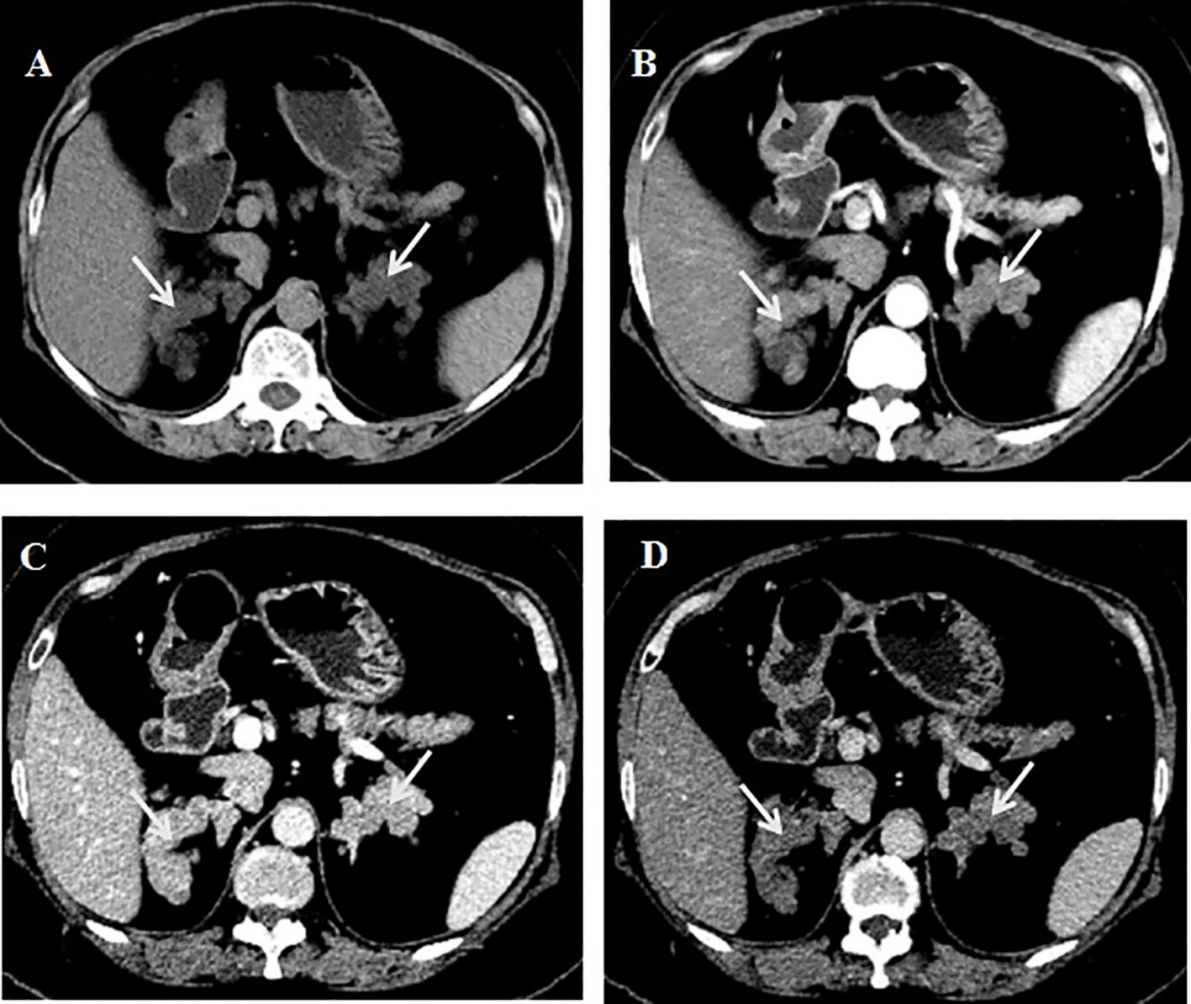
CT of adrenal gland. (A) Plain scan.(B) Enhanced scan arterial phase. (C) Enhanced scan portal phase. (D) Enhanced scan delayed phase.
